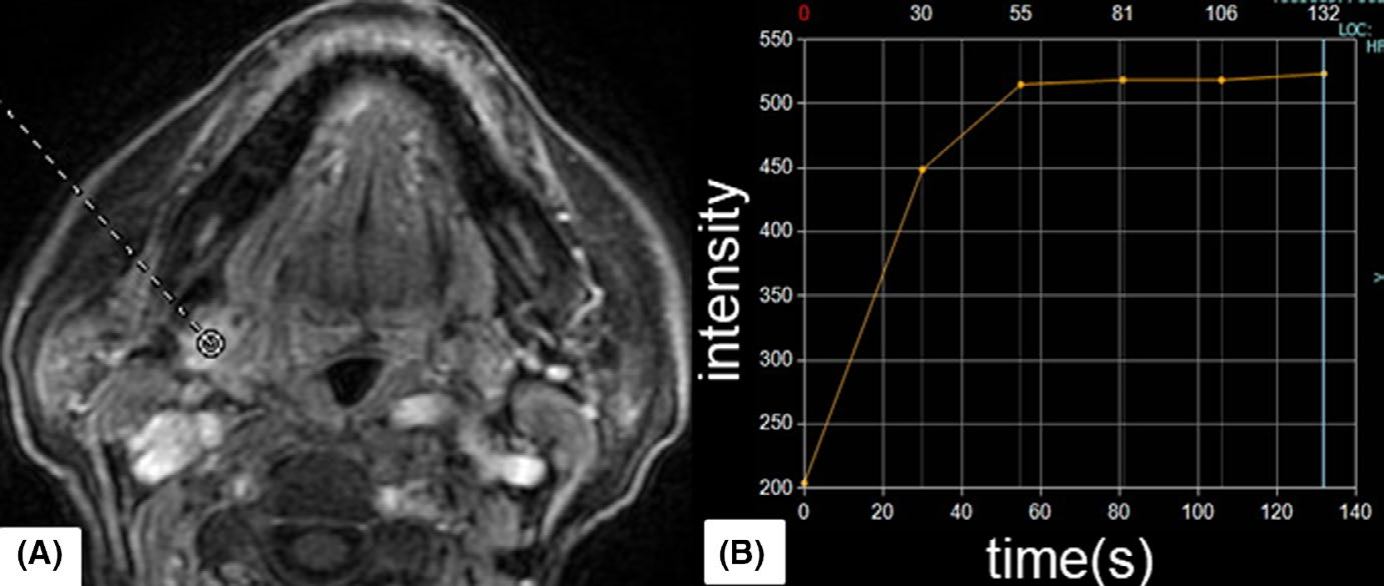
A, Selected area for time‐signal intensity curve (TIC) of dynamic contrast‐enhanced MRI is shown with dotted circle. B, TIC shows a plateau enhancement pattern (type‐C), peak time <120 s, and wash‐out ratio <30%
